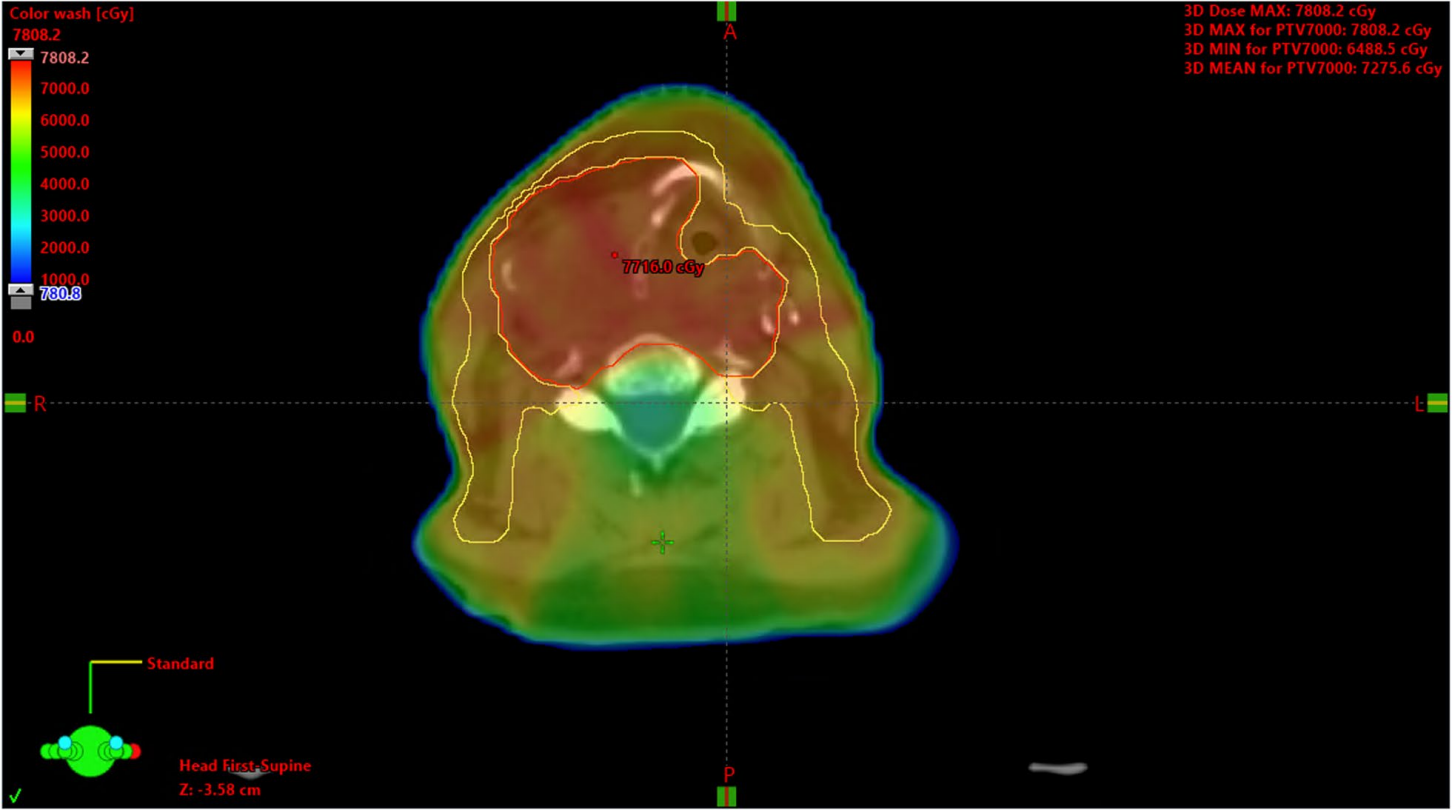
Axial view of patient’s CT-based radiation treatment plan. 6  MV photon beams were utilized, and patient was prescribed to 7000 cGy in 35 fractions to the isocenter. Dose color wash is represented as a percentage relative to the prescribed 7000 cGy dose. Thyroid mass contours are delineated in red and are treated to 7000 cGy, while retropharyngeal and cervical neck nodal levels IB, II, III, and IV are delineated in yellow and treated to 6300 cGy
